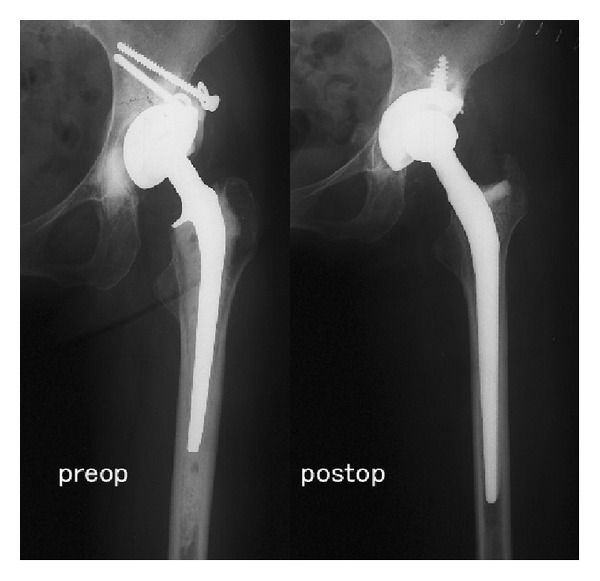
A 54-year-old woman underwent primary THA for osteoarthritis of the hip. Eleven years later, revision THA was performed using the ROBODOC system due to aseptic loosening. Full weight bearing was possible within 1 week after surgery. At the latest follow-up, 9 years postoperatively, no clinical or radiographic problems were identified.
